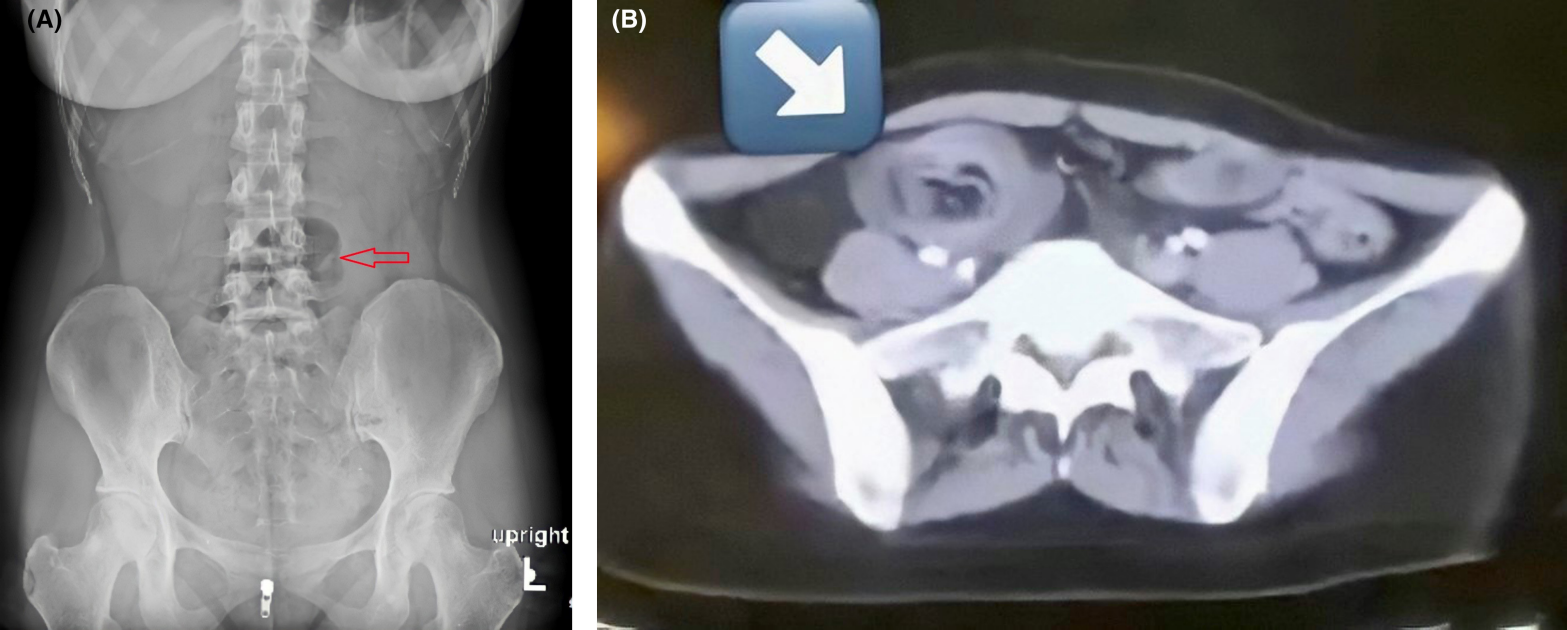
(A) Plain abdominal radiography suggests intestinal obstruction (arrow). (B) CT scan of the abdomen; Target sign appearance at the right side of the abdomen (arrow)
